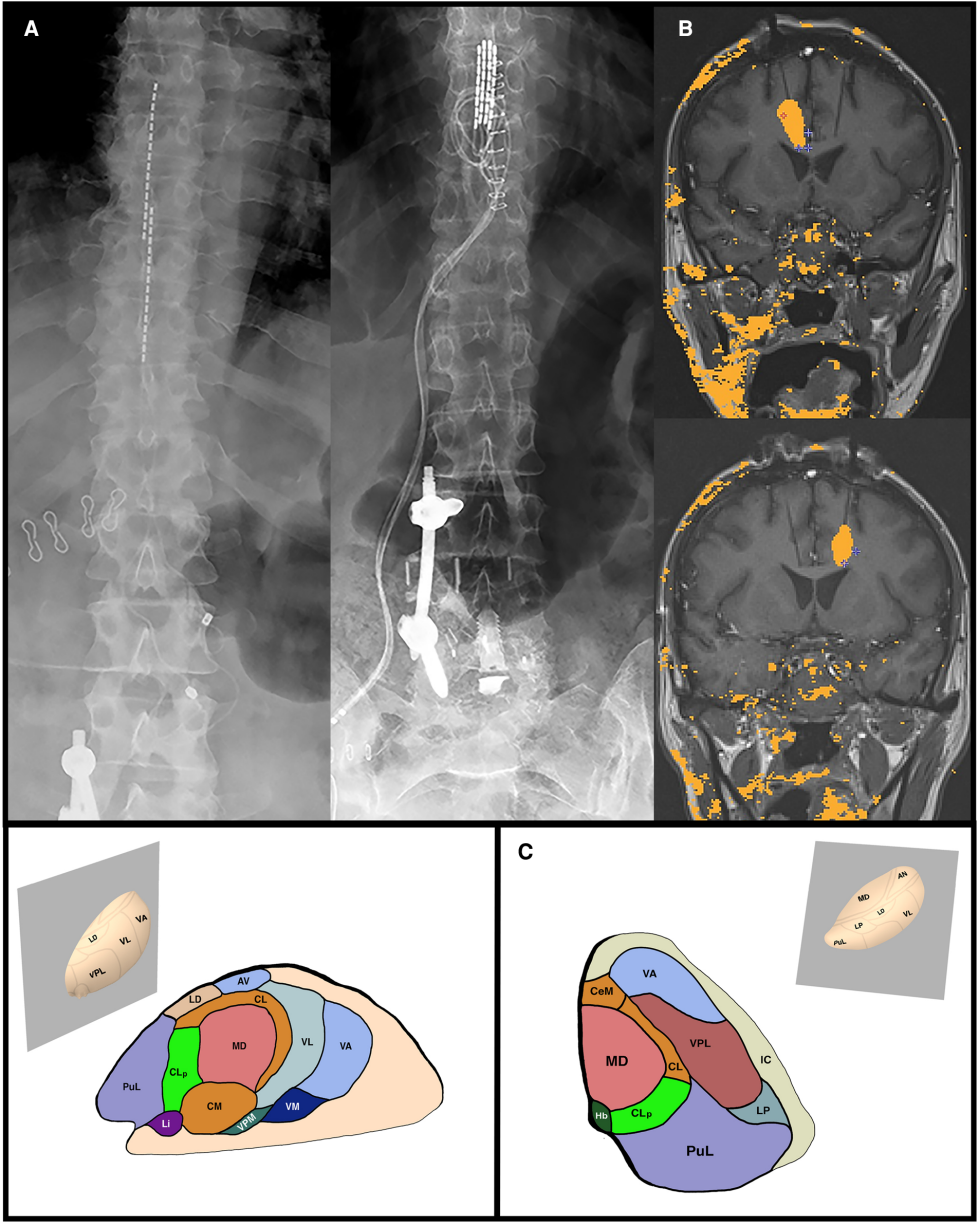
(A) Examples of percutaneous SCS leads (LEFT) and paddle SCS leads (RIGHT). (B) Intraoperative coronal MRI thermometry images of the completed right (TOP) and left (BOTTOM) anterior cingulotomy laser-induced thermal lesions. (C) Thalamic anatomy. Sagittal and Axial planes of the thalamus through the CLp. AV, anterior ventral nucleus; CM, centre median nucleus, CeM, central medial nucleus; CL, central lateral nucleus; CLp, posterior central lateral nucleus; Hb, habenular nucleus; LD, lateral dorsal nucleus; Li, limitans nucleus; LP, lateral posterior nucleus; MD, mediodorsal nucleus; PuL, pulvinar; VA, ventral anterior nucleus; VL, ventral lateral nucleus; VM, ventral medial nucleus; VPM, ventral posterior medial nucleus; VPL, ventral posterior lateral nucleus.
